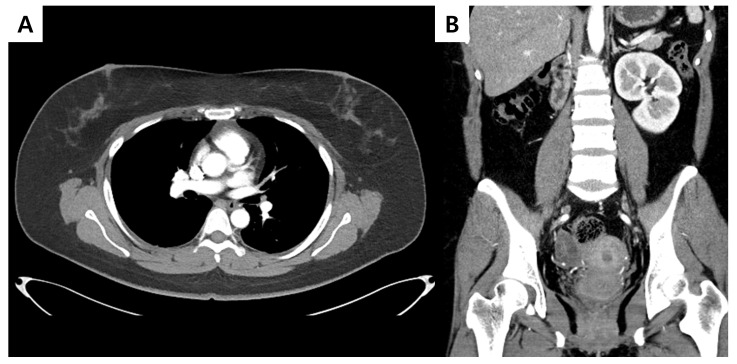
ChestCT obtained 3 months after surgery demonstrated no imaging findings suspicious for tumor recurrence or metastatic disease, including the bilateral breasts (A), and abdominal CT showed no evidence of another primary site or metastatic disease involving the gynecologic organs (B). Subsequent follow-up Chest and abdominal CT examinations performed at 13, 23, 35, and 47 months after surgery also revealed no evidence of recurrence or metastatic disease.
